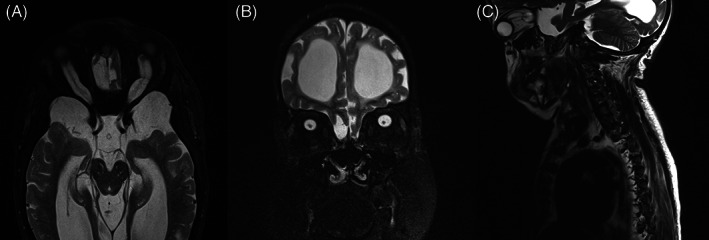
T2‐fat saturation MRI for patient 3 showing exuberant enlargement of subarachnoid space with “sausage” sign in axial section and reduced thickness of optic nerves, more pronounced on the left. (A) Axial section; (B) coronal section; (C) sagittal section
